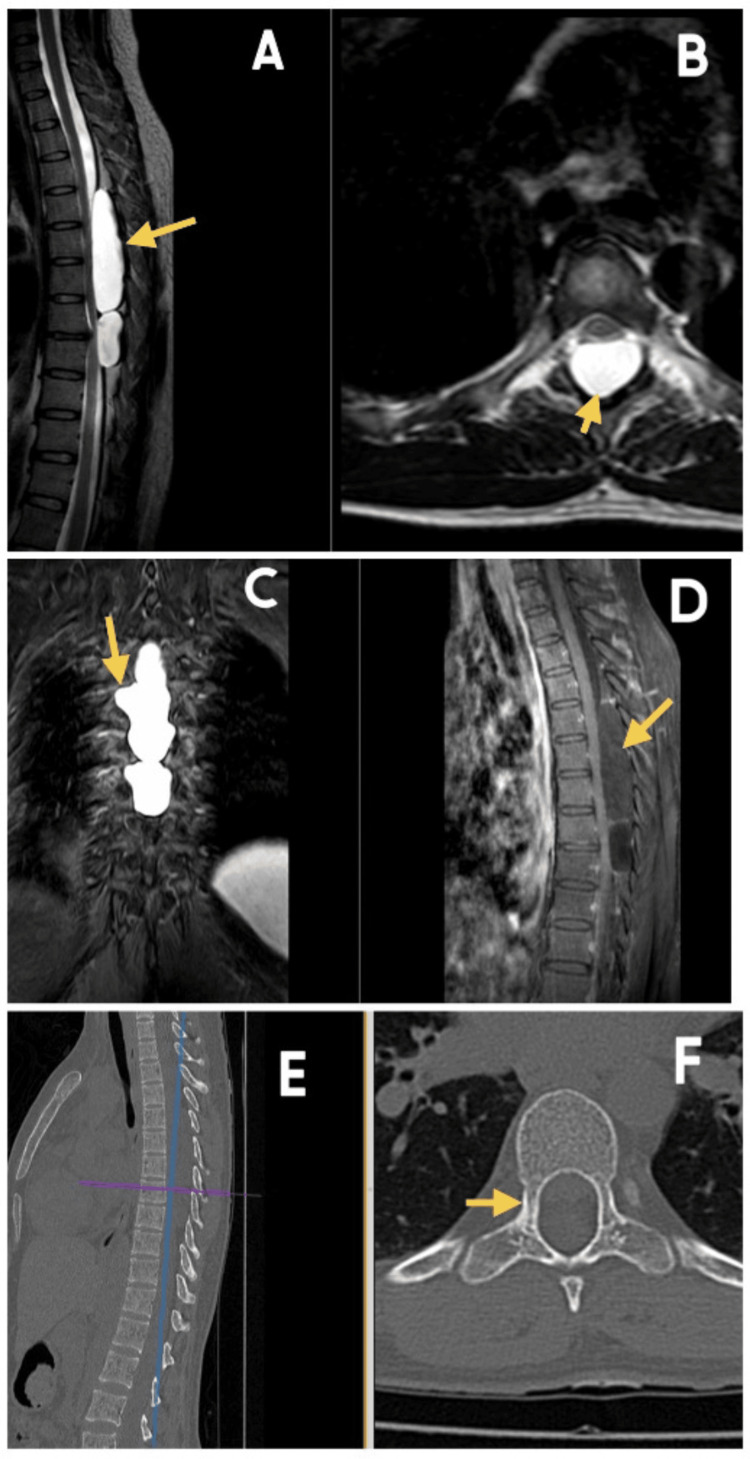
Preoperative imaging (A) T2-weighted sagittal MRI showing two well-defined large extradural hyperintense lesions causing compression over the underlying cord. (B) T2-weighed axial section. (C) Coronal T2-weighted MRI showing lateral extension of the arachnoid cyst into the foramen. (D) Contrast MRI sequence showing no postcontrast enhancement. (E) Sagittal CT scan of the dorsal spine. (F) Axial CT of the thoracic spine showing widening of the spinal canal and thinning of the pedicles.
